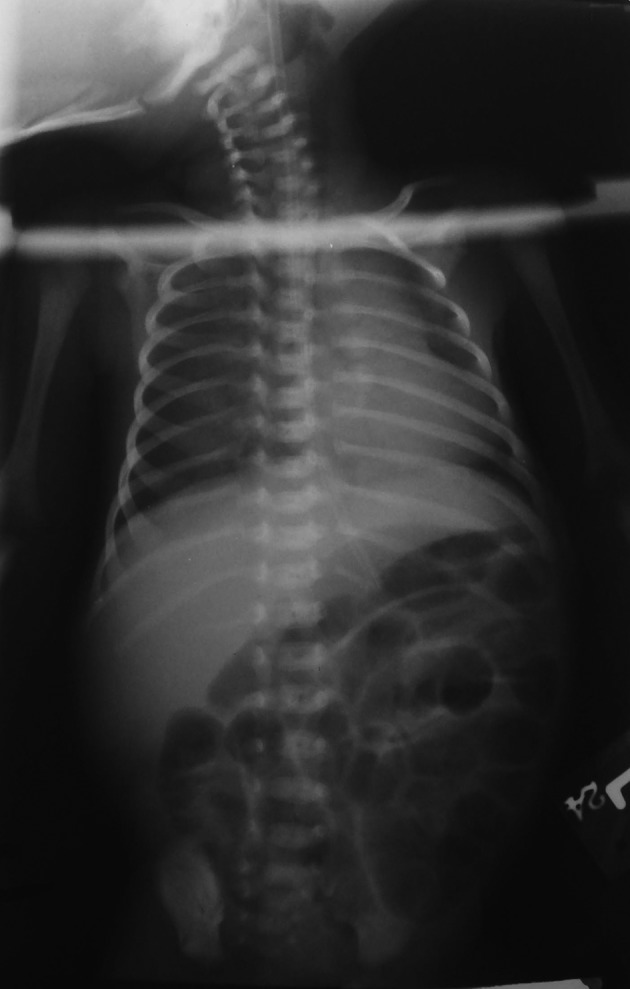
Chest and abdomen plain Radiograph
